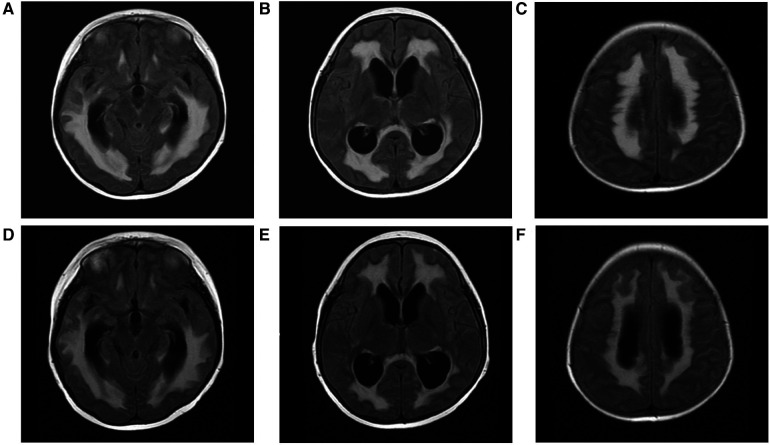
T2-FLAIR hyperintensity contrast-enhanced lesions, multiple abnormal signals in bilateral cerebral white matter after haematopoietic stem cell transplantation (A–C). Decreased area of white matter lesions after treatment (D–F).
